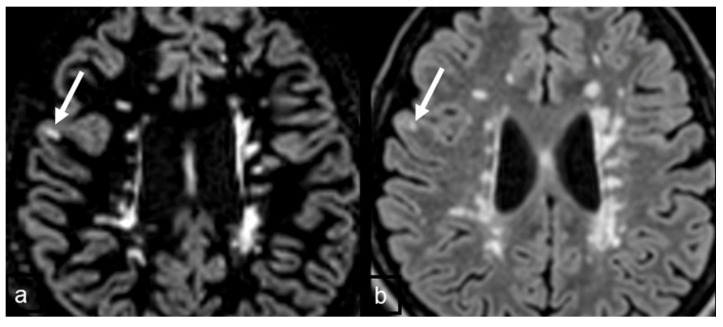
The right frontal juxtacortical lesion (arrow) is detected both with DIR (a) and with FLAIR images (b); however, it is more evident on DIR. Note that DIR and FLAIR images are similar in detection of periventricular lesions.
